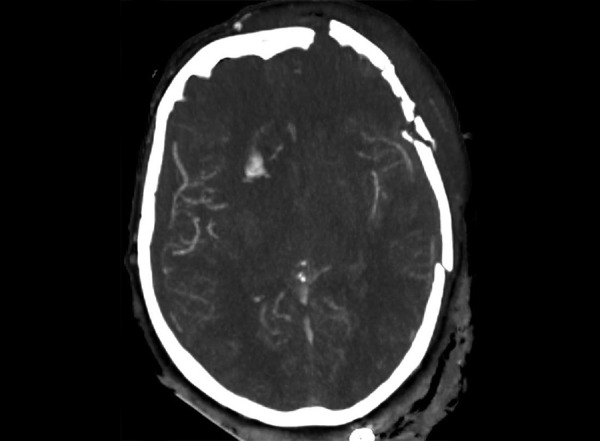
Axial head CT scanner with angiography showing a right anterior cerebral artery rupture with free contrast extravasation
